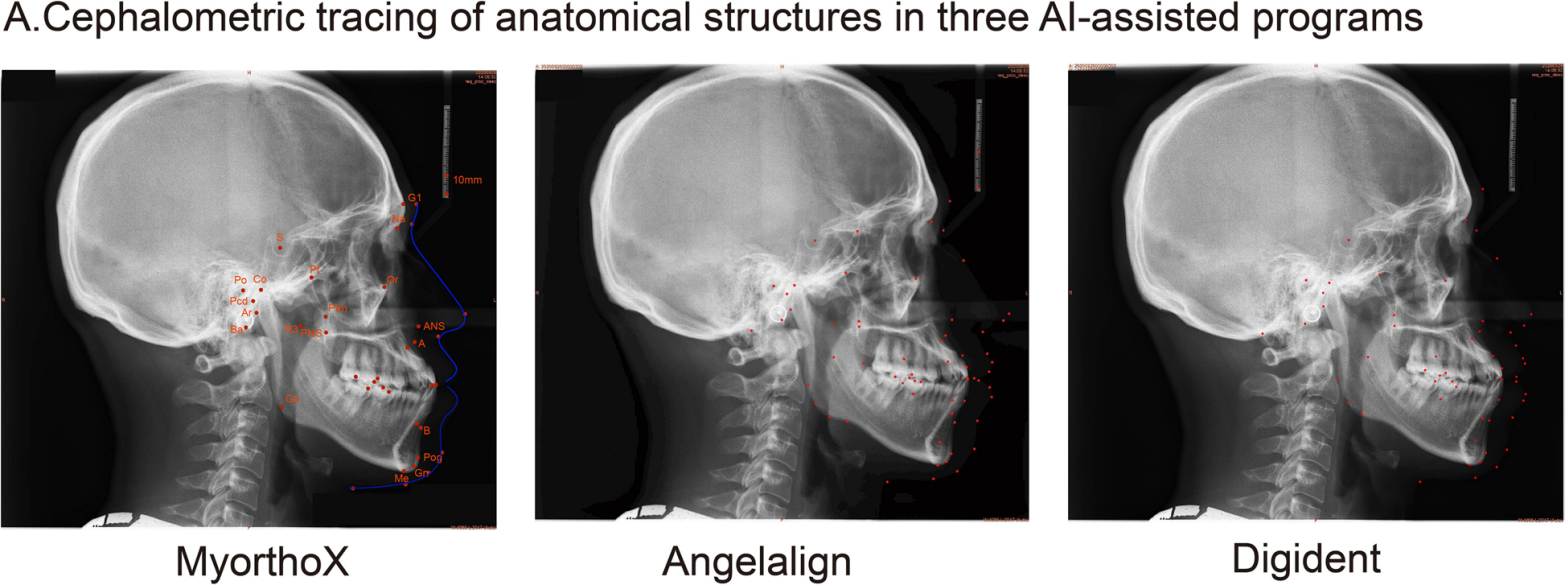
Cephalometric tracing of anatomical structures in three AI-assisted programs. Sample lateral cephalometric radiograph with a 30-mm ruler uploaded to the MyOrthoX, Angelalign, and Digident programs
